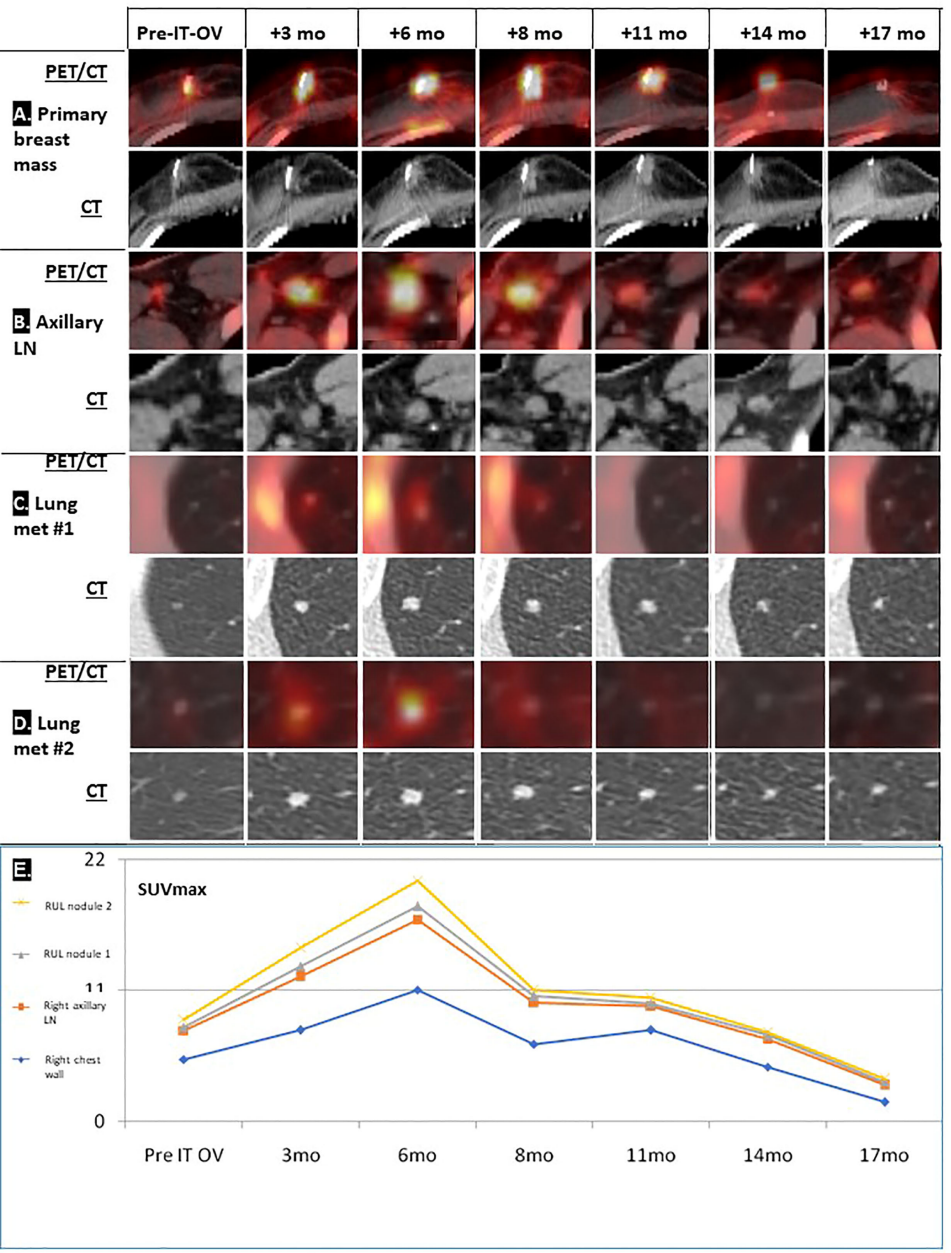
Radiological follow-up of breast lesion and metastases following IT-OV immunotherapy. PET/CT and CT images over the 17 months of treatment of four primary breast masses (A) and axillary lymph nodes (B) where intratumoral oncolytic virotherapy was administered, and two lung metastases (C, D). Graphic summary of chronological radiological pattern of hyperimmune response in the right chest wall, right axillary lymph node (LN) and right upper lung (RUL) nodules 1 and 2, as measured by PET (SUV_max)_ (see Figure 2) (E).
